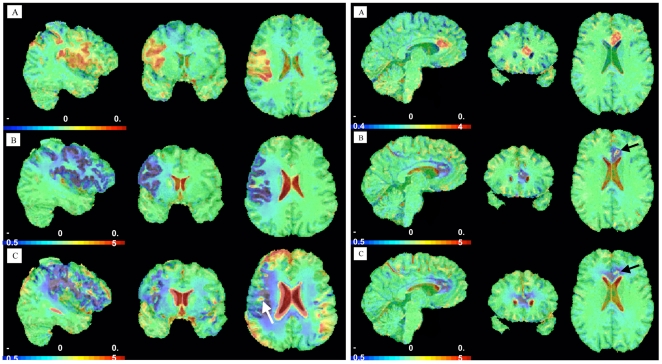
VGM volume fields in Patients 12 (left) and 13 (right). Left: The first MRI, obtained within 48 hours after stroke onset, is compared to MRIs obtained 4 days (A), 3 months (B), and 5 years (C) later. After 5 years, volume increases can be detected in a spotlike distribution (arrow) within the infarcted area. Right: Volume fields are shown from MRIs obtained 5 days (A), 3 months (B), and 5 years after the first MRI had been obtained immediately after stroke onset. A small area of volume increase (arrow) within the original ischemic zone, first seen after 3 months, still persists.
